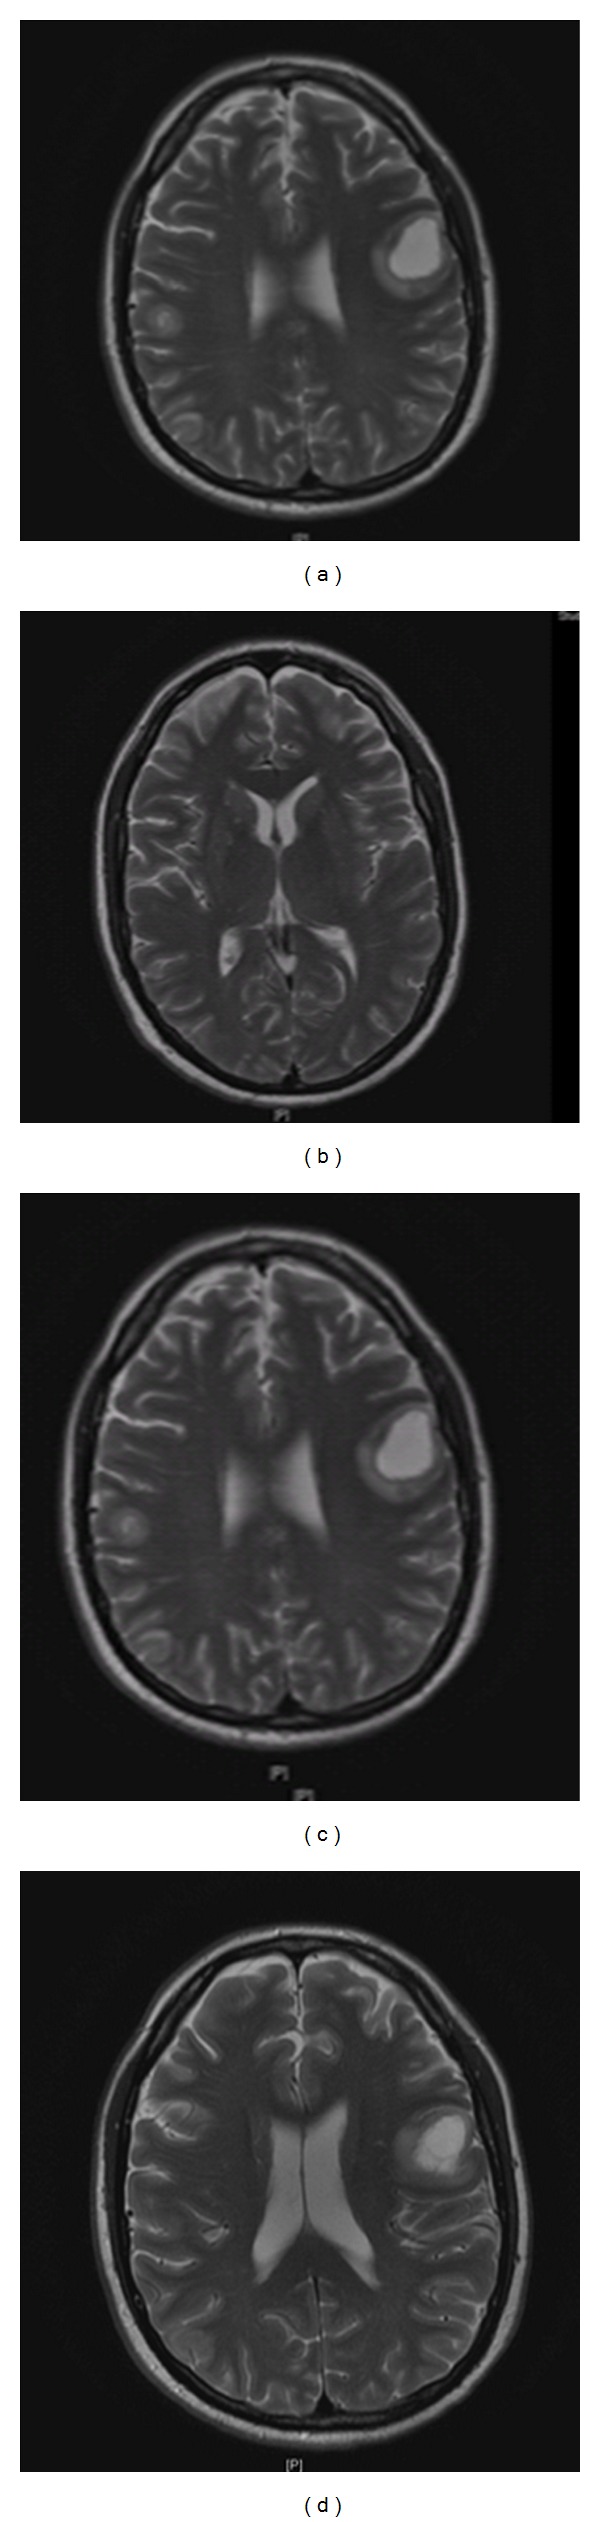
Magnetic resonance imaging of head showing multiple new acute hemorrhages in gray-white junction, mainly seen in the intra- and supratentorial space, measuring 2 cm or less.
